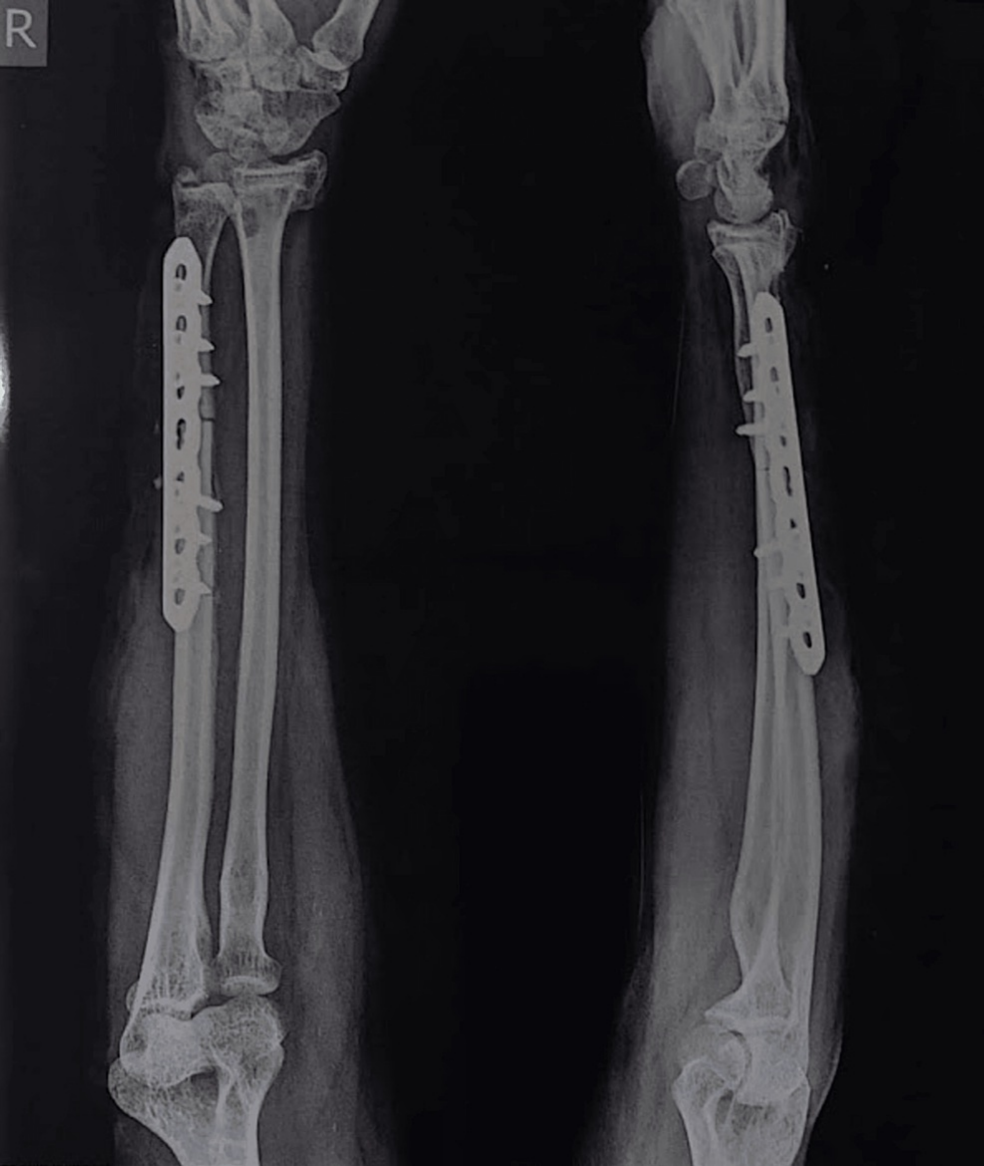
Post-operative radiograph
